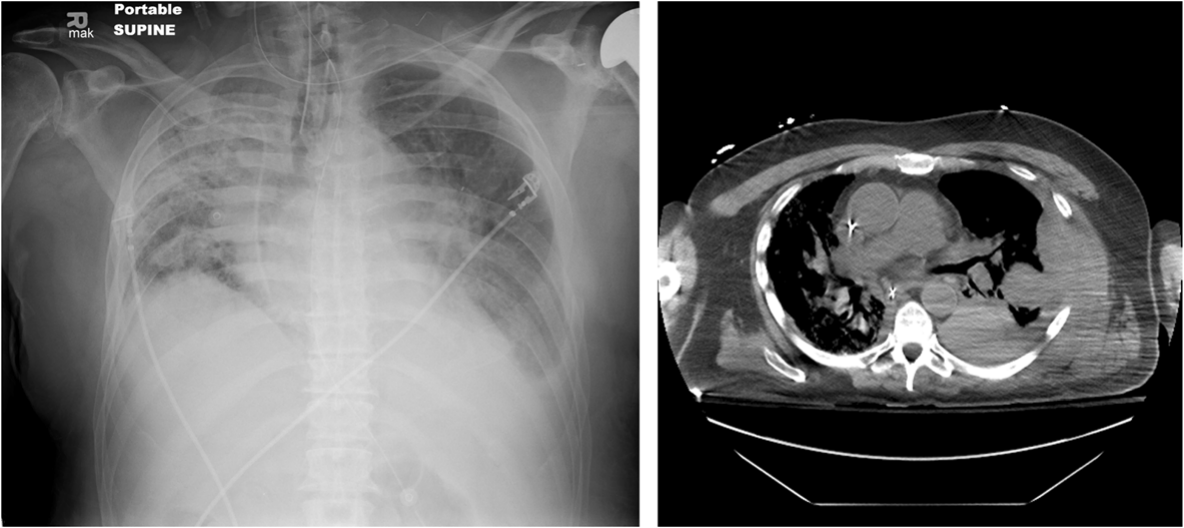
Chest radiograph and CT scan of a patient with left hemothorax resulting in hemodynamic instability
